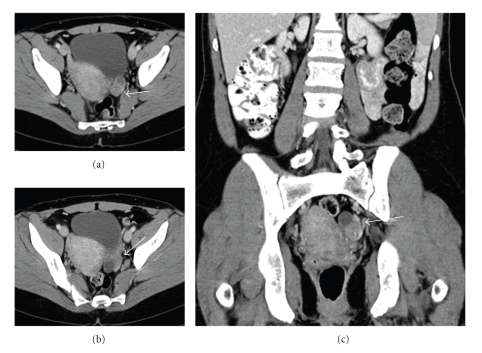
CT scan of the abdomen and pelvis (10/13/2007). Axial (a) and (b) and Coronal (c) images obtained through the pelvis demonstrate a complex solid and cystic mass in the left adnexa (whie arrow) measuring 3.2 × 2.4 cm.
